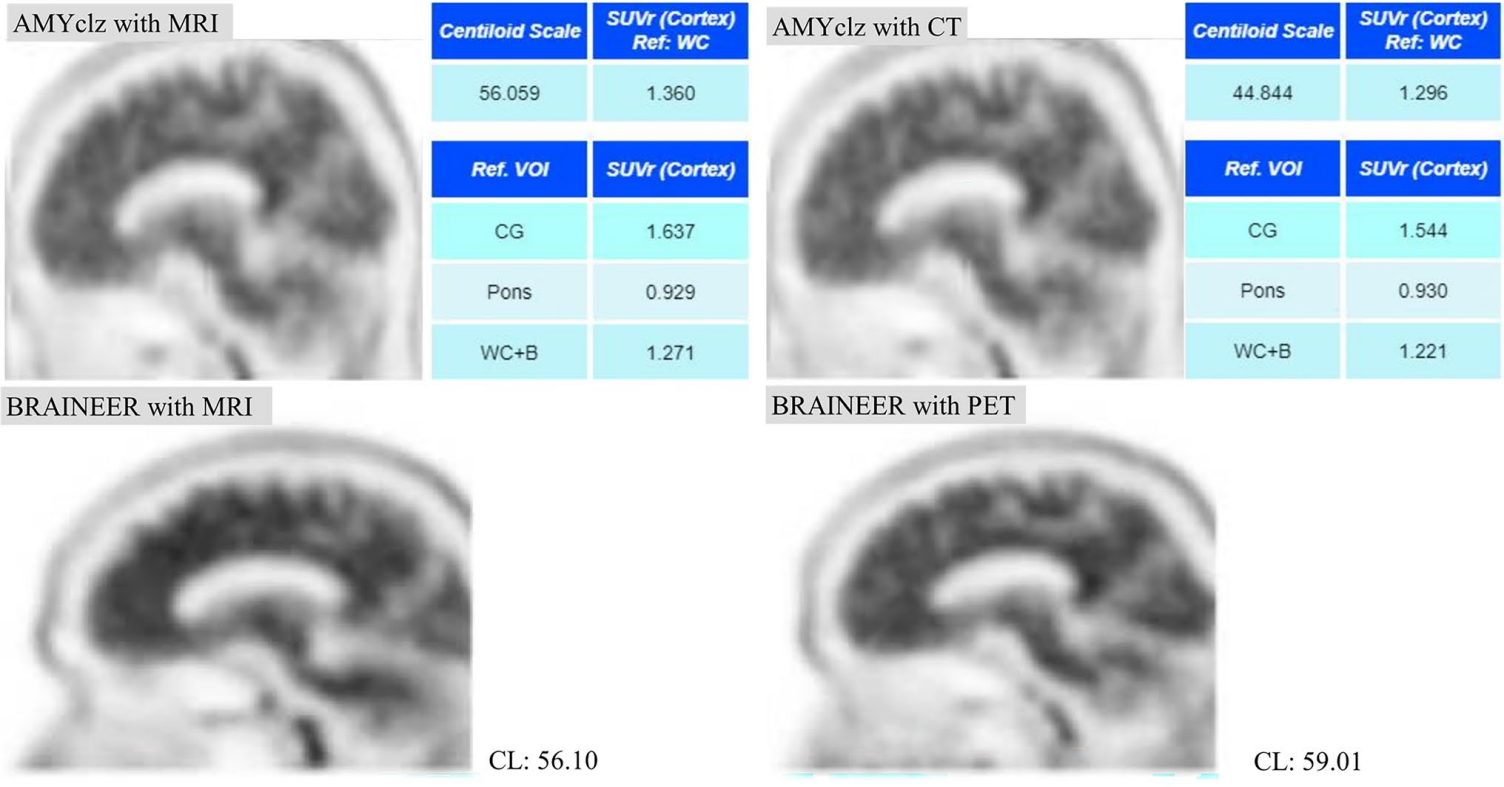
Analysis results by software for the same case, using anatomically standardized PET images and AMYclz^®^ Neuro. Following anatomical standardization, CT-based imaging showed slightly higher cerebellar uptake than MRI-based imaging in this case. Considering the unchanged SUVr in the pons, it was presumed that cerebellar uptake was elevated on CT-based imaging
